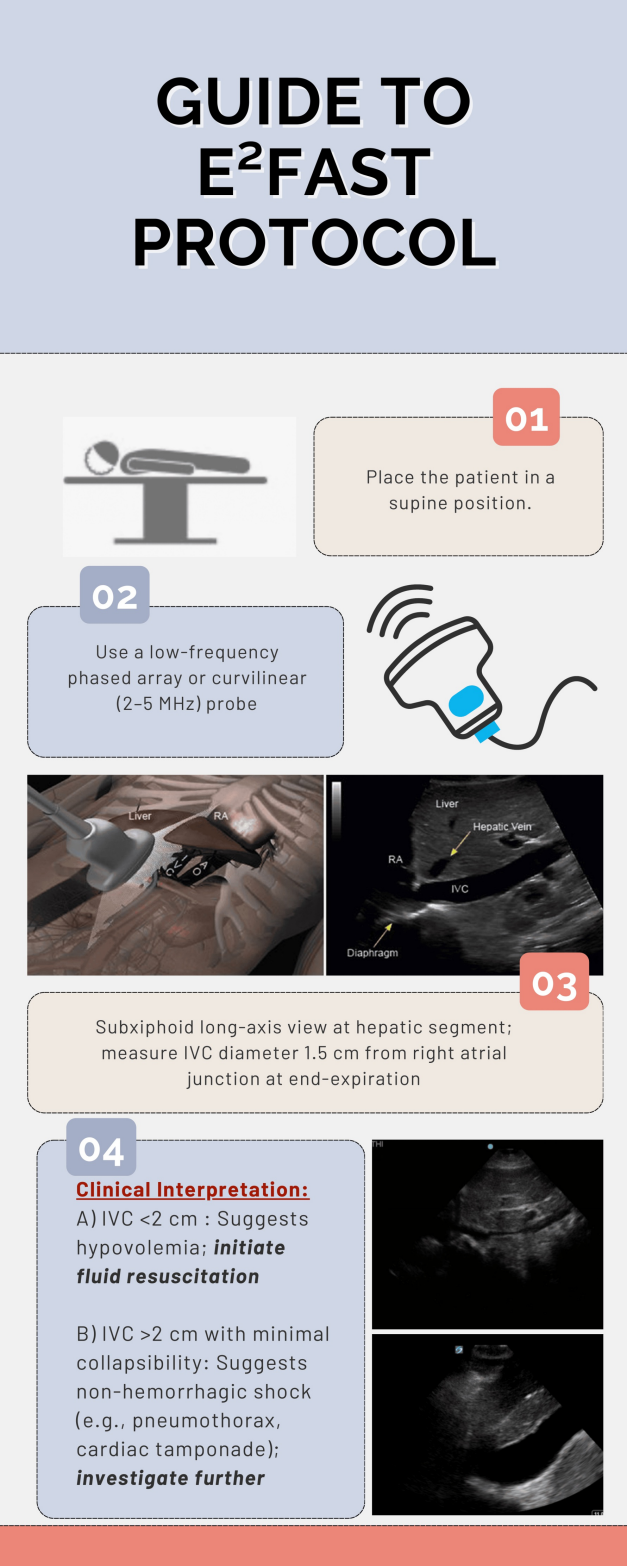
Proposed standardized protocol of e²FAST. Adapted from “The ICU Ultrasound Pocket Book,” Killu et al. [25]. The main author granted permission. IVC = inferior vena cava; RA = right atrium
